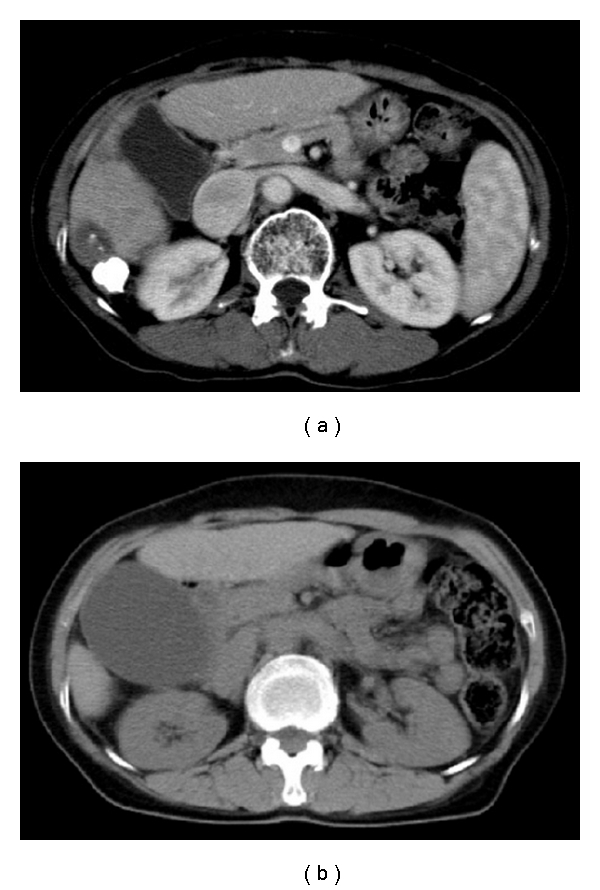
(a) Gallbladder swelling cannot be seen on baseline abdominal CT before sorafenib administration. (b) A highly tense and enlarged gallbladder can be seen on abdominal CT. There is no thickening of the gallbladder wall. The intrahepatic and common bile ducts are not dilated, and there are no calculi in the gallbladder or tumorous lesions in the neck of the gallbladder.
